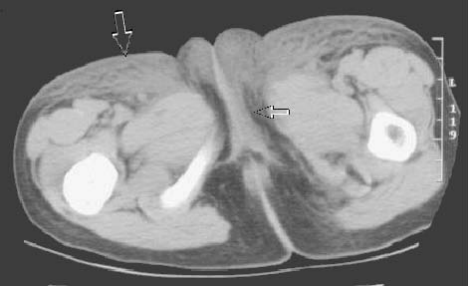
Labial edema. Unenhanced axial computed tomography image depicts extensive bilateral subcutaneous edema (large arrow) with extension into the labia (small arrow).
